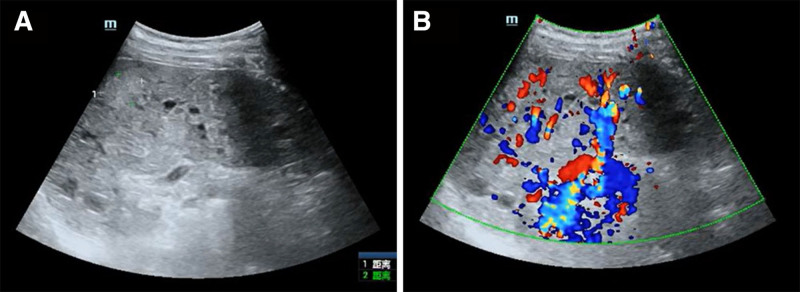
The ultrasound indicates splenomegaly, widespread high-density nodular lesions within the spleen, and a strong likelihood of vascular or lymphatic lesions.
